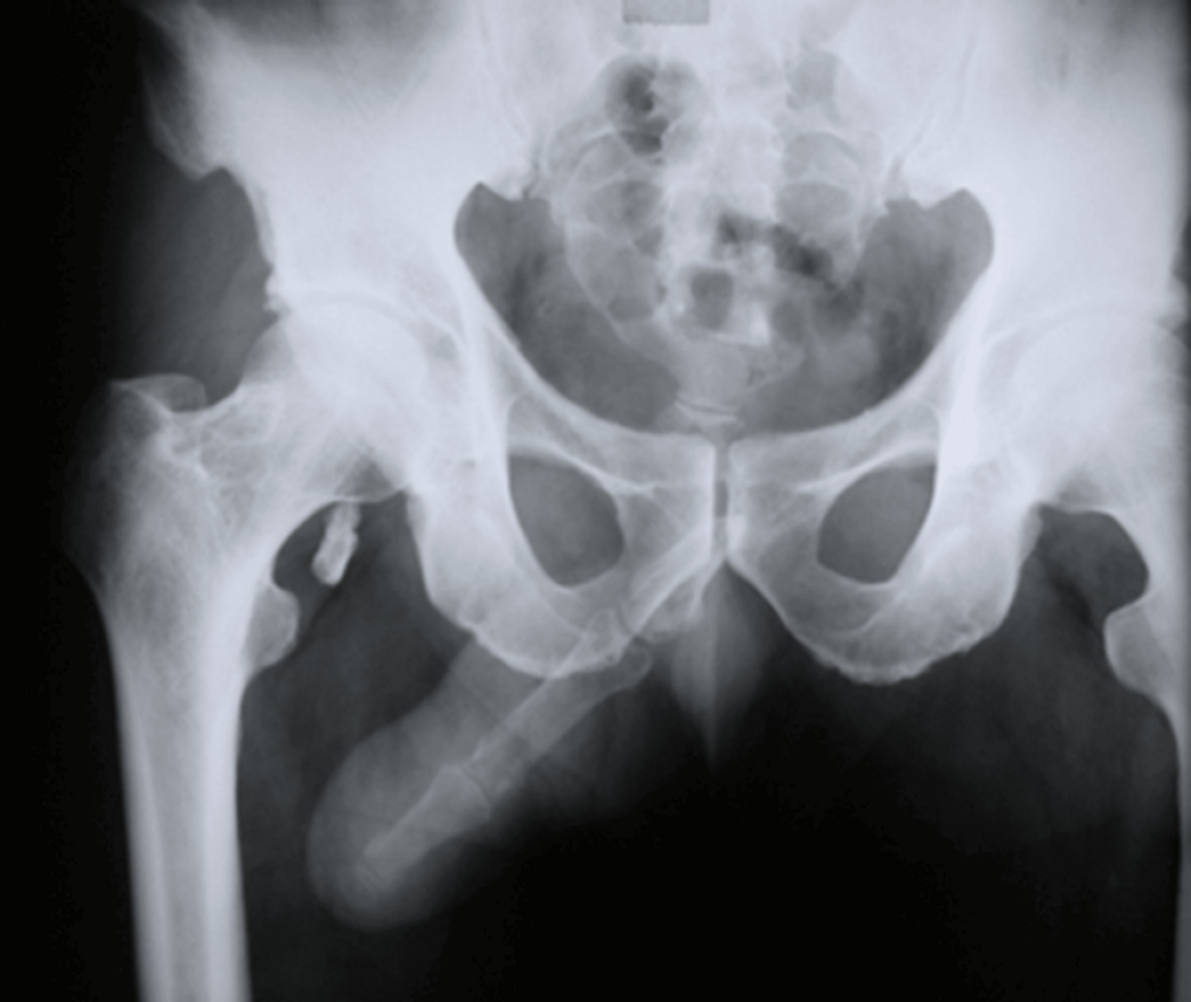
X-ray ten years post-surgery. No phalangeal bone resorption was noted, indicating an adequate blood supply.
